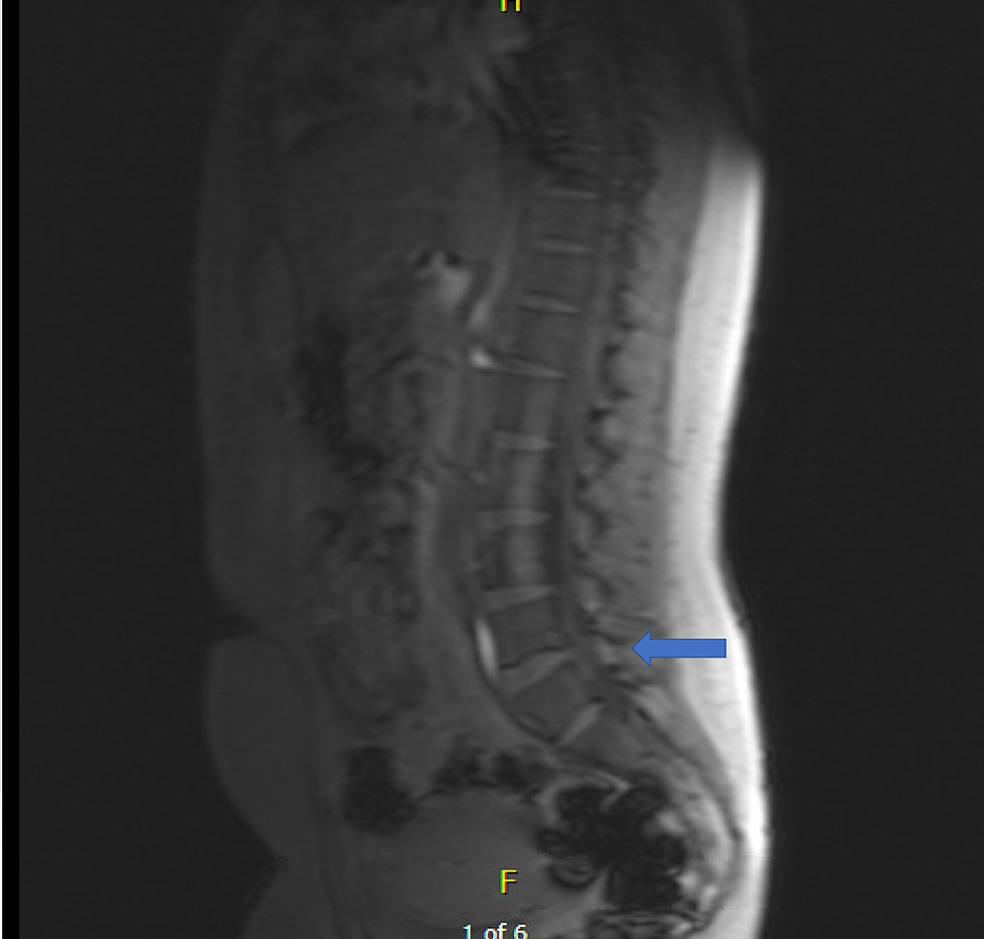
MRI lumbar spine sagittal view MRI lumbar spine sagittal view showing L4-5 disk degeneration with mild spinal stenosis (blue arrow)
